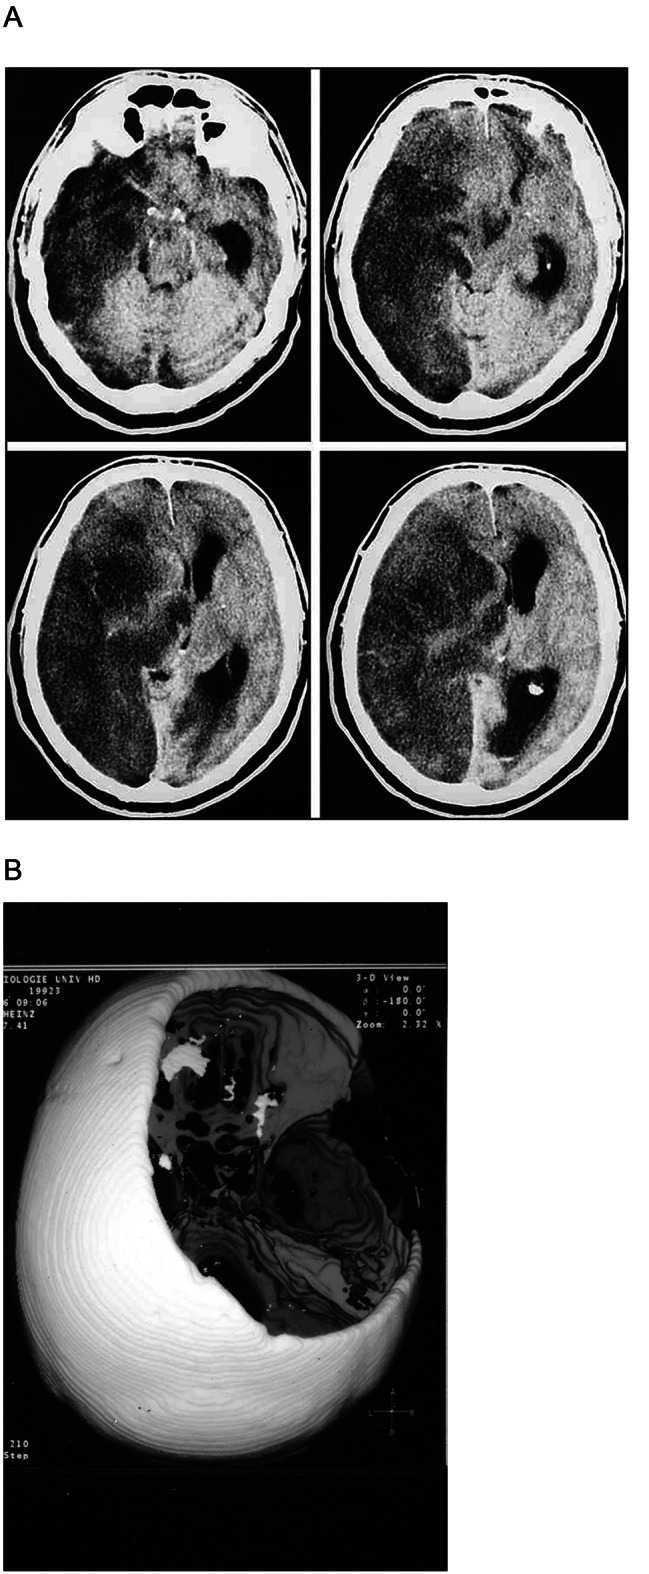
(A) From the original publication of Hacke et al. [60] (52). Non-contrast computed tomographic scans of a 56-year-old man 34 h after onset of severe right-sided hemiparesis. The midline shift was measured with a ruler on the individual images and converted to the true dimensions; the midline shift was 12 mm. The patient had a unilateral fixed, dilated pupil. He died 12 h later. (B) 3-dimensional model of hemicraniectomy indicating the necessary size for bone removal
